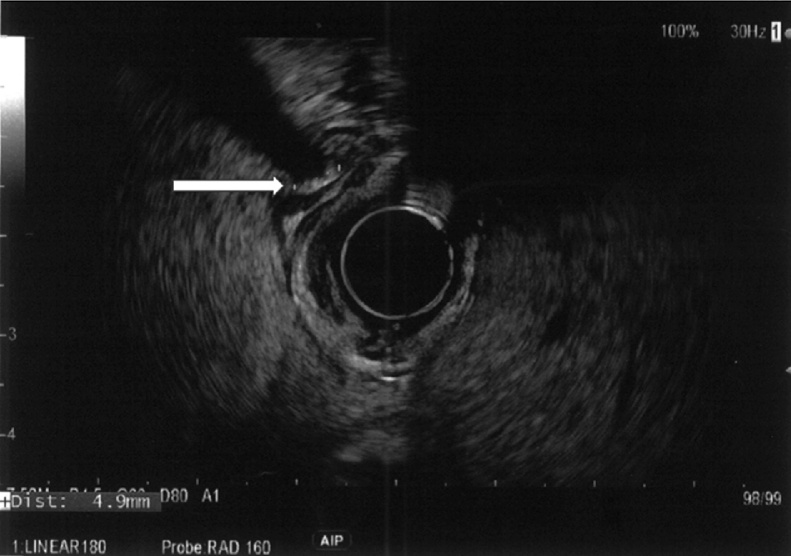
Endoscopic ultrasound showing a 4.9 mm gallstone and acoustic shadow (arrow) in the common bile duct.
